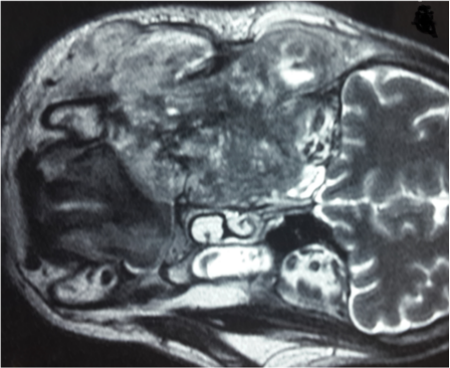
Coronal T2-weighted magnetic resonance image of the facial area showing the ethmoidonasal, and intraorbital extension, with infiltration of the jugal and temporozygomatic soft parts
